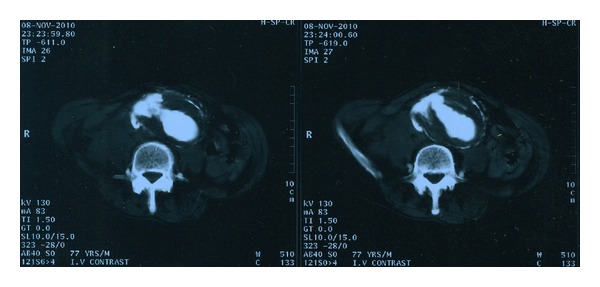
Abdominal CT identifying a large 7.8 cm AAA with evidence of retroperitoneal rupture. Notice the synchronous contrast filling of the aorta and IVC and their communication through the sac, indicating aortocaval fistulation.
